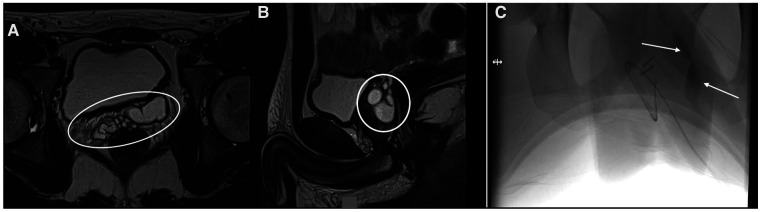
(A,B) MRI showing the SVC (white arrows); (C) genitography performed using a 3Ch ureteral catheter inserted into the left ejaculatory duct contrasting the left SVC.
